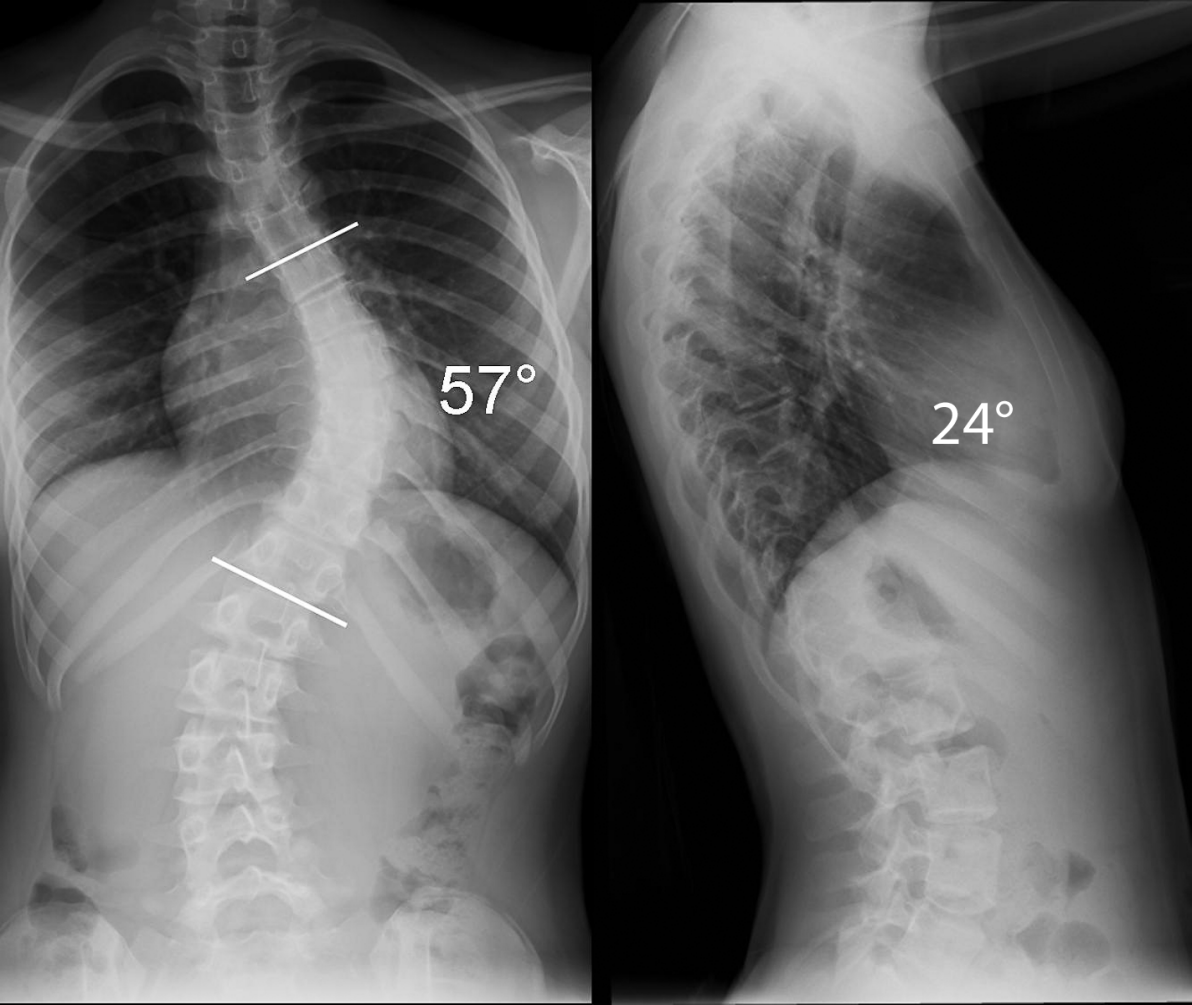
"Patient 2 - Anteroposterior and lateral preoperative radiographs." Anteroposterior and lateral radiographs show a 57° main thoracic curve which was corrected using the MIS technique. A slightly different pattern of pedicle screws was utilized in this patient, as shown in Figure 14 & 15.
